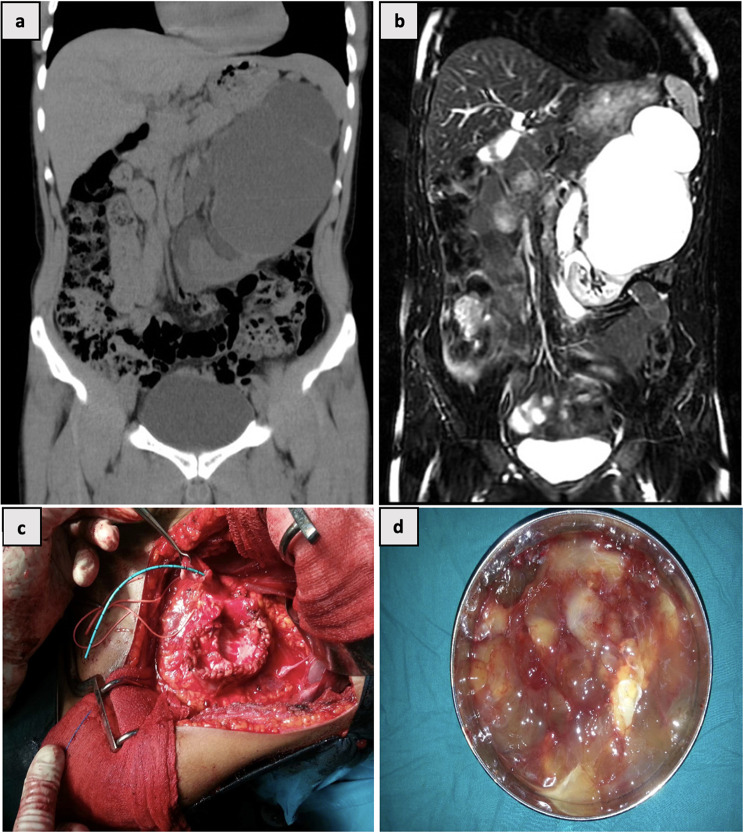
(a) Plain CT KUB showing a duplex left collecting system with gross hydronephrosis of left upper moiety (b) MR Urogram T2 images showing duplication of collecting system on left side with two ureters noted in proximal & middle 1/3rd and gross dilatation of upper moiety PCS and ureter (c) Intra op image of upper moiety nephrectomy (d) Thick Mucus drained from upper moiety
